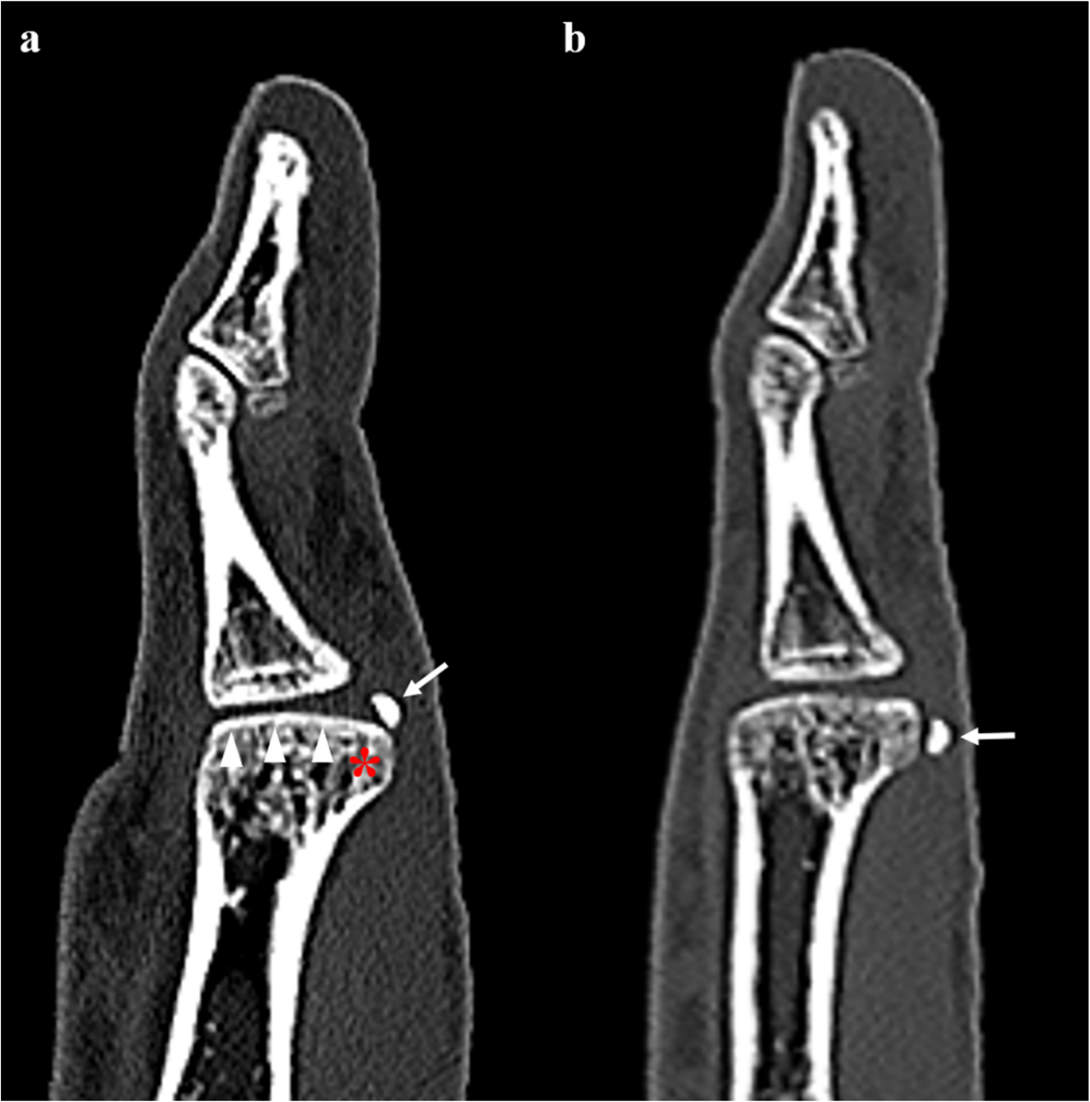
Sagittal reformation image of computed tomography (a) shows proximal displacement of the radial sesamoid (arrow). Note the non-round (flat) articular surface (arrow heads), prominence of the radial condyle of the metacarpal head (asterisk), and sharp proximal edge of the radial sesamoid. After reduction, the radial sesamoid (arrow) has relocated (b)
